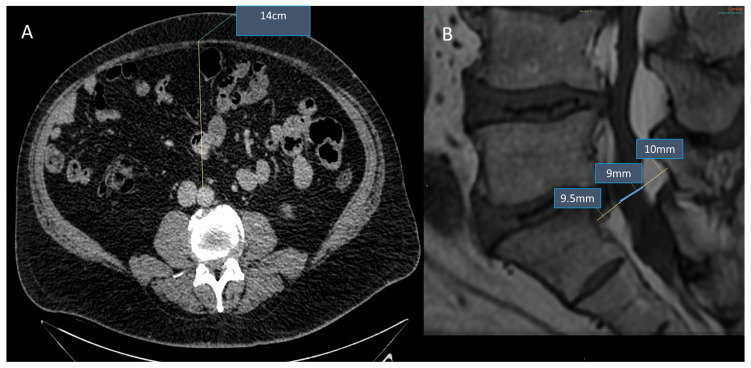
Obese patient (45 years old, male, BMI = 31 kg/m^2^, dyslipidemia). Association between SEL and visceral fat deposition: CT of the abdomen (Panel A) reveals increased visceral fat deposition (AP diameter 14 cm), while lumbosacral MRI sagittal T1w (Panel B) reveals SEL grade 2: moderate (dural-sac/epidural fat index = 0.46).
